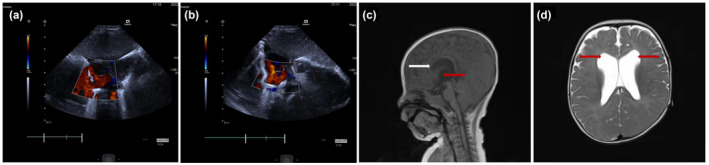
Clinical features. (a,b) Color Doppler echocardiography showing lac of closure of the foramen ovale; the procedure was interrupted at the foramen ovale of the atrial septum, with multiple strands of left to right septal blood were seen in the middle of the atrial septum, the widest of about 3 mm (10 days old). (c) Cranial MRI at 7 months old showing enlargement of the third ventricle (red arrow) and thinning of the corpus callosum (white arrow). (d) Bilateral ventricular enlargement (red arrow)
